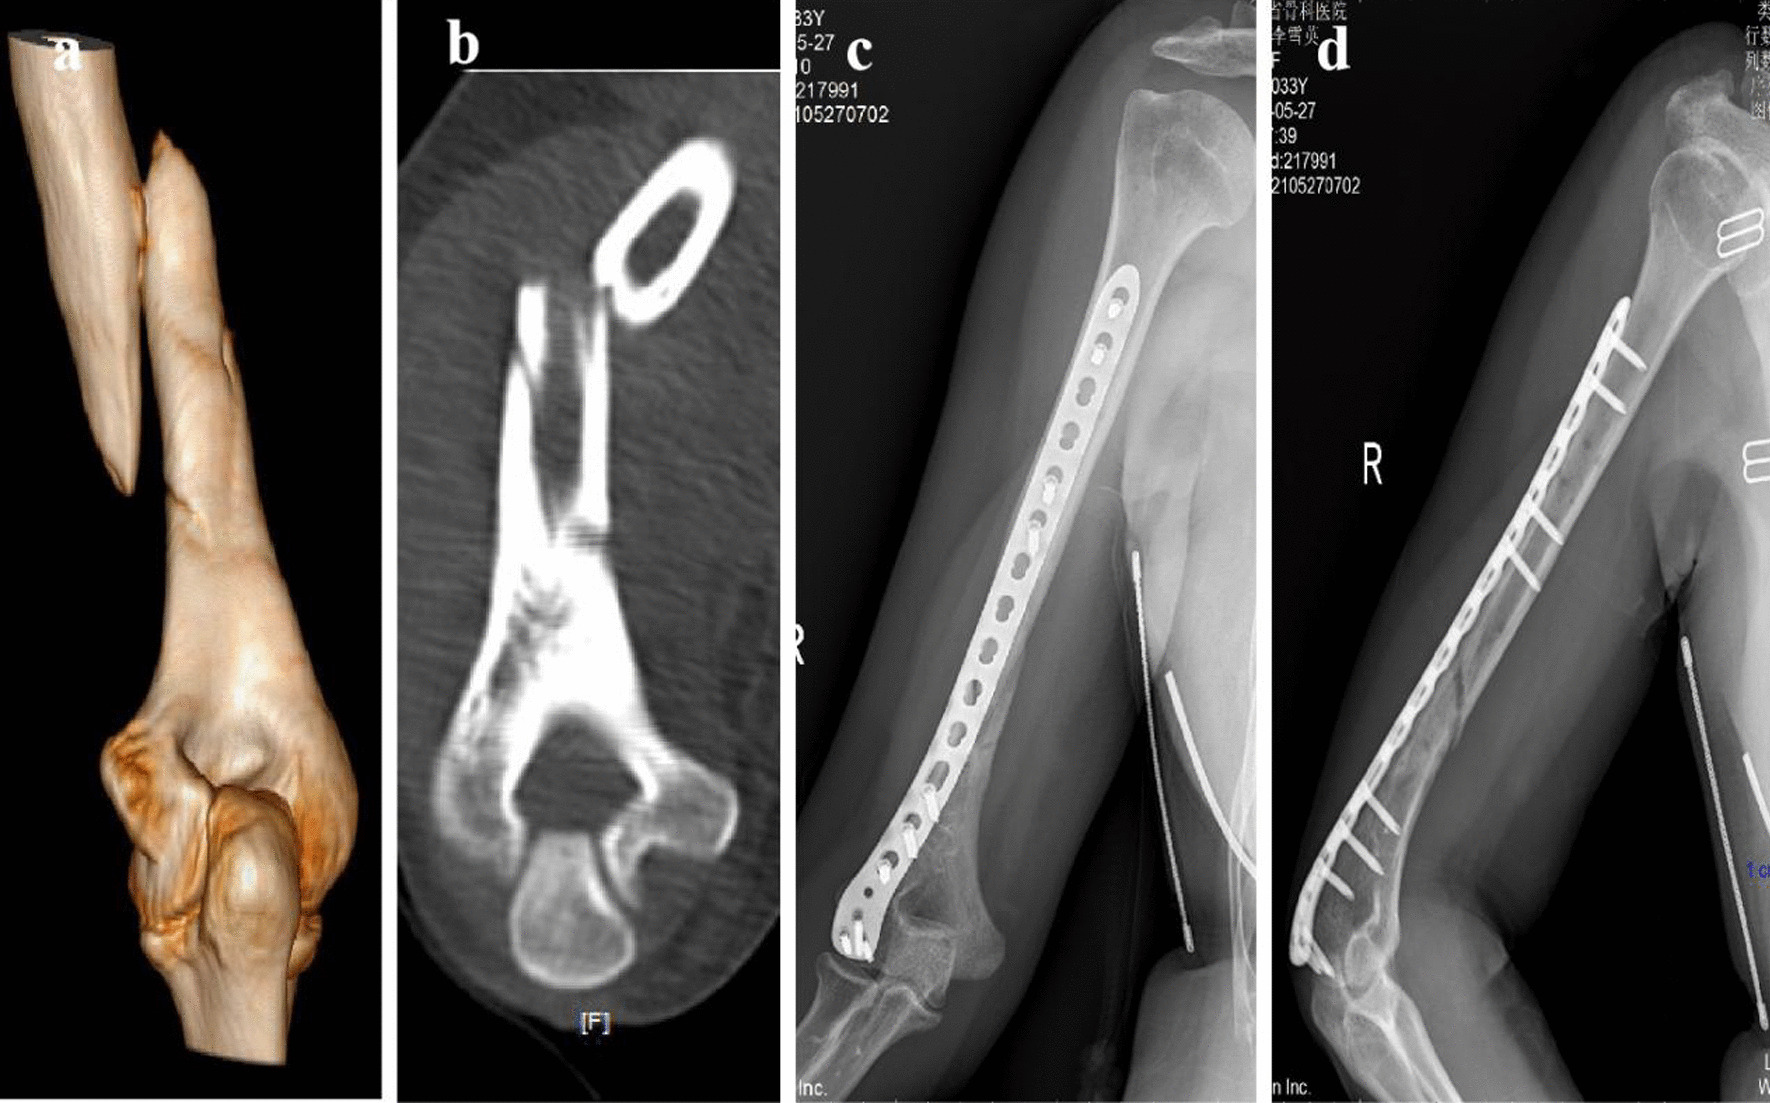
The MIPPO used in Case 2: a 34-year-old male, indirect violence in a fall leads to the right middle-inferior humerus fractures. a Preoperative 3-D CT imaging of the middle-inferior humerus fractures. b Preoperative CT coronal imaging of the middle-inferior humerus fractures. c The anteroposterior X-ray radiograph of the full-length humerus 2 months after MIPPO. d The lateral X-ray radiograph of the full-length humerus 2 months after MIPPO
